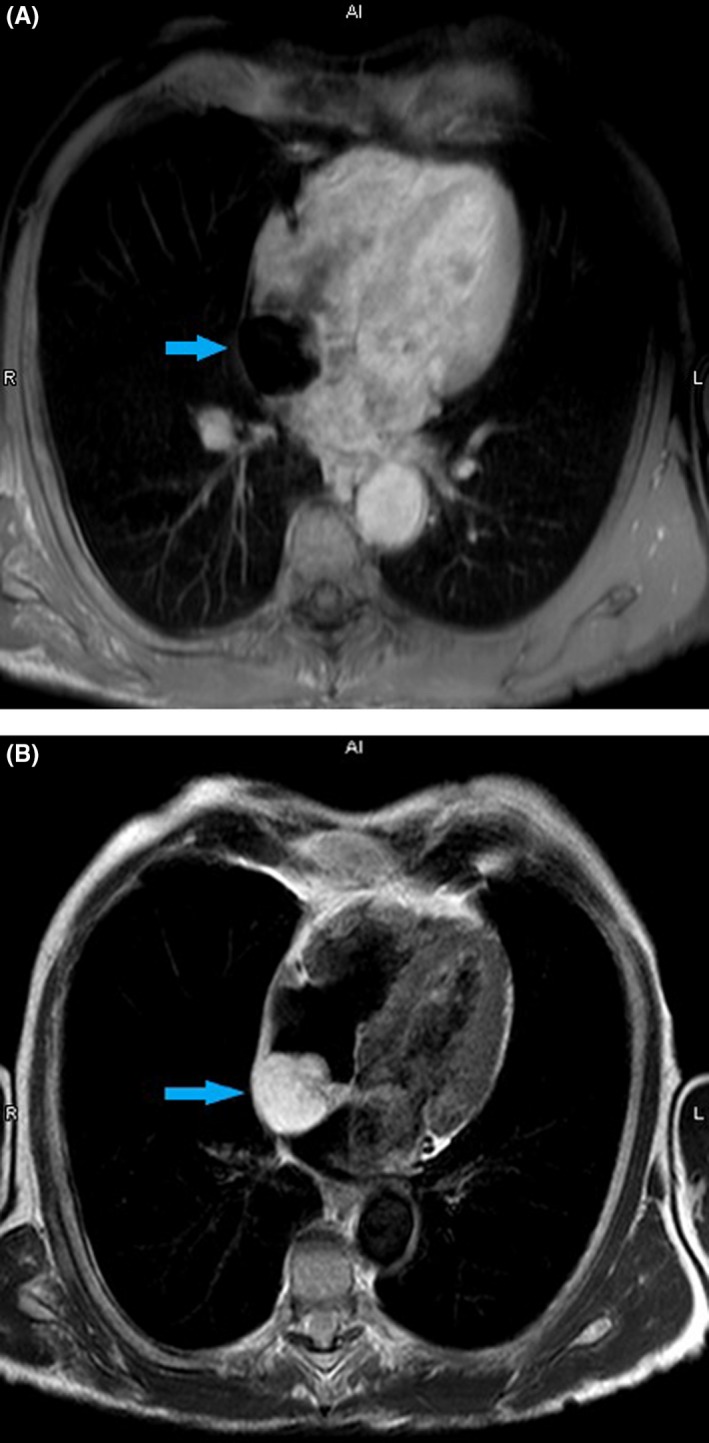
A, Arrow showing cardiac lipoma—T1 weighted sequence in Cardiac MRI. B, Arrow showing cardiac lipoma—T2 weighted sequence in Cardiac MRI
